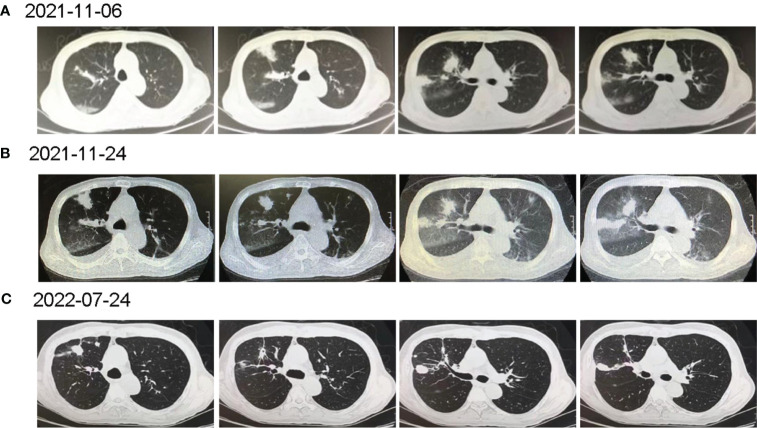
The computed tomography diagnosis of admitted patient. (A) Chest CT revealed multiple inflammations in both lungs, with localized pulmonary solidity and a few foci of fibroproliferation in the anterior segment of the upper lobe of the right lung; (B) Chest CT (15 days post-treatment) showed increased exudative lesions in both lungs and right pleural effusion; (C) Chest CT (8 months post-treatment) revealed that the lesions in both lungs were significantly reduced.
